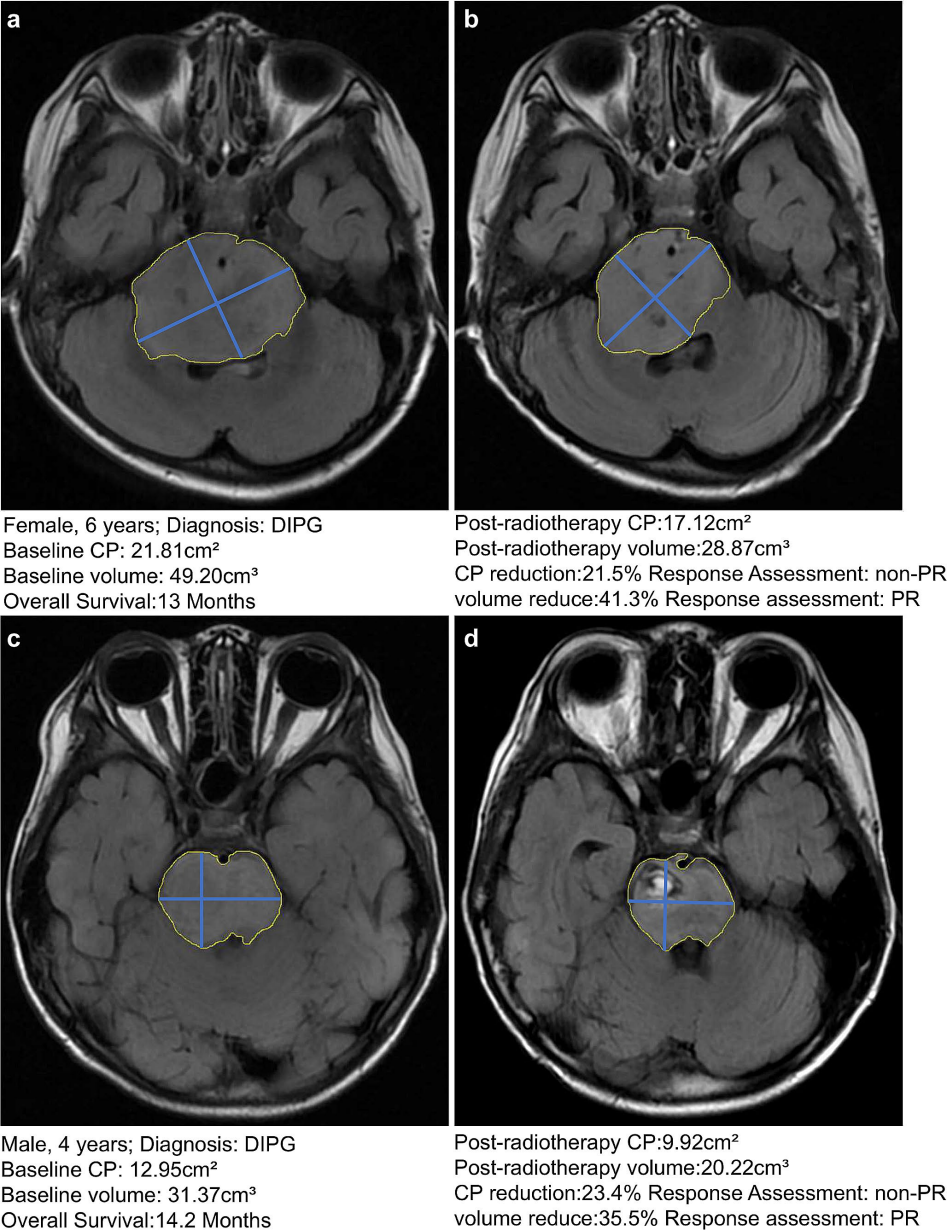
Representative case of PR with a discordant classification based on a CP reduction of 25% and volume reduction of 32%. (a-b). A 6-year-old female DIPG patient with an overall survival (OS) of 13 months. Measurement of the 2D product of the maximum peripheral diameter and tumor volume at baseline and post-radiotherapy in T2-FLAIR images. The CP and volume measurements showed that the tumor exhibited a 21.5% CP reduction and 41.3%, volume reduction post-radiotherapy. Non-PR and PR classifications based on CP and volume response assessments, respectively. (c-d). A 4-year-old female DIPG patient with an overall survival (OS) of 14.2 months. Measurement of the 2D product of the maximum peripheral diameter and tumor volume at baseline and post-radiotherapy in T2-FLAIR images. The CP and volume measurements showed that the tumor exhibited a 23.4% CP reduction and 35.5% volume reduction post-radiotherapy. Non-PR and PR classifications based on CP and volume response assessments, respectively DIPG, diffuse intrinsic pontine glioma; FLAIR, fluid-attenuated inversion recovery; CP, cross-product; PR, partial response; 2D, two-dimensional
